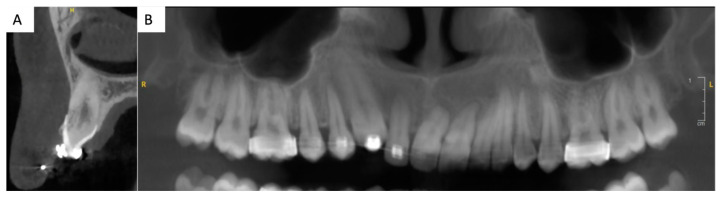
Three-dimensional imaging, CBCT (cone beam computed tomography): (A): sagittal section of tooth 13. Thin buccal and palatal cortical bone plates and proximity of the apex of 13 with the right nasal cavity can be noted. (B) Two-dimensional reconstruction of the upper jaw. Important root resorptions of the central maxillary incisors can be noted. A root resorption of the lateral incisor is also present.
